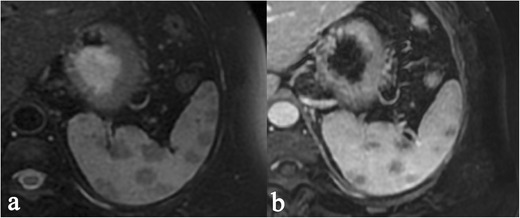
MRI appearance of a splenic sarcoidosis (same patient as Fig. 2). The visualization of the nodules reaches an optimal level on T2-weighted acquisition with fat suppression (Fig. 5a) and in the early gadolinium-enhanced T1 images (Fig. 5b)
